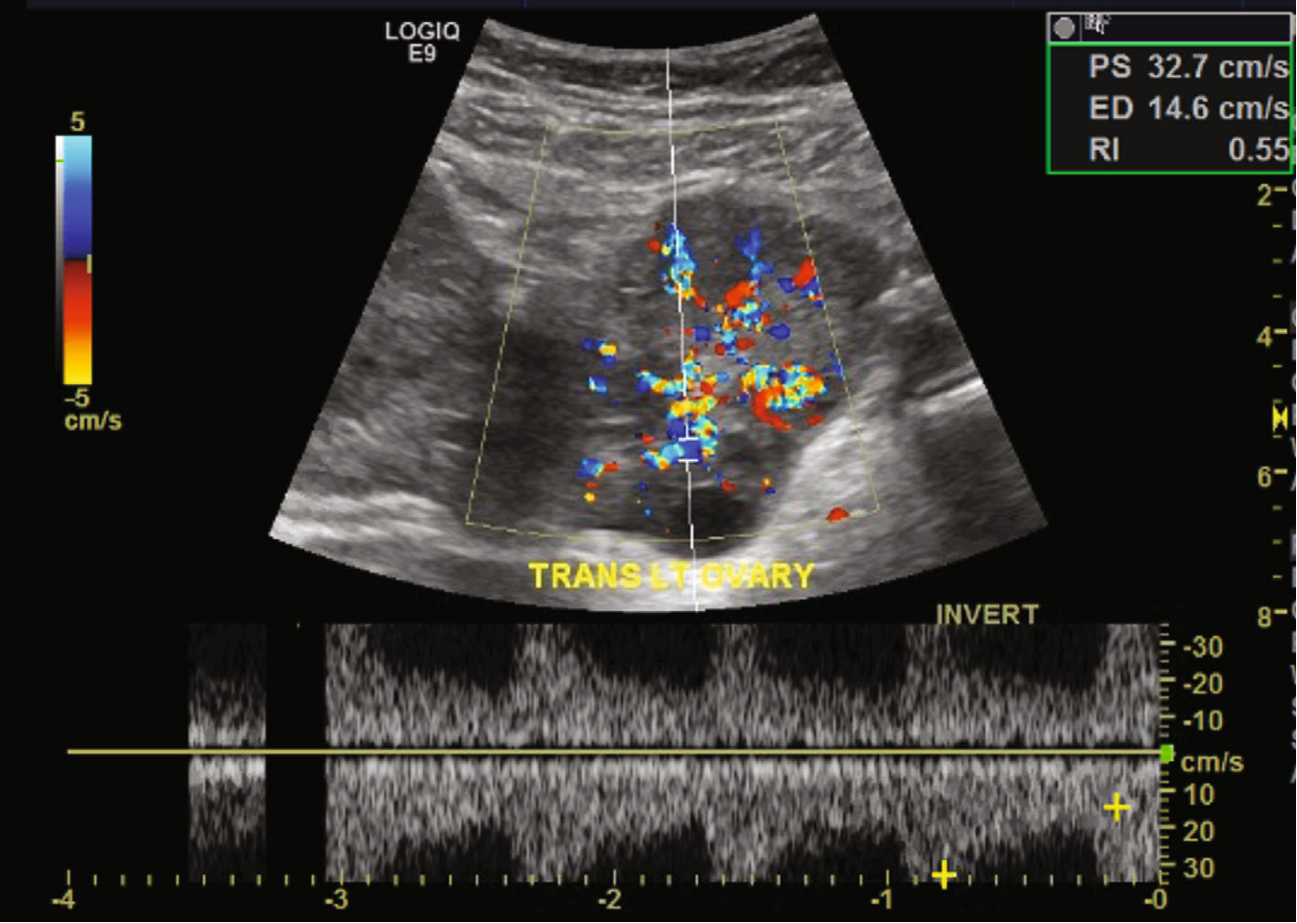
Left adnexa with Doppler flow.
